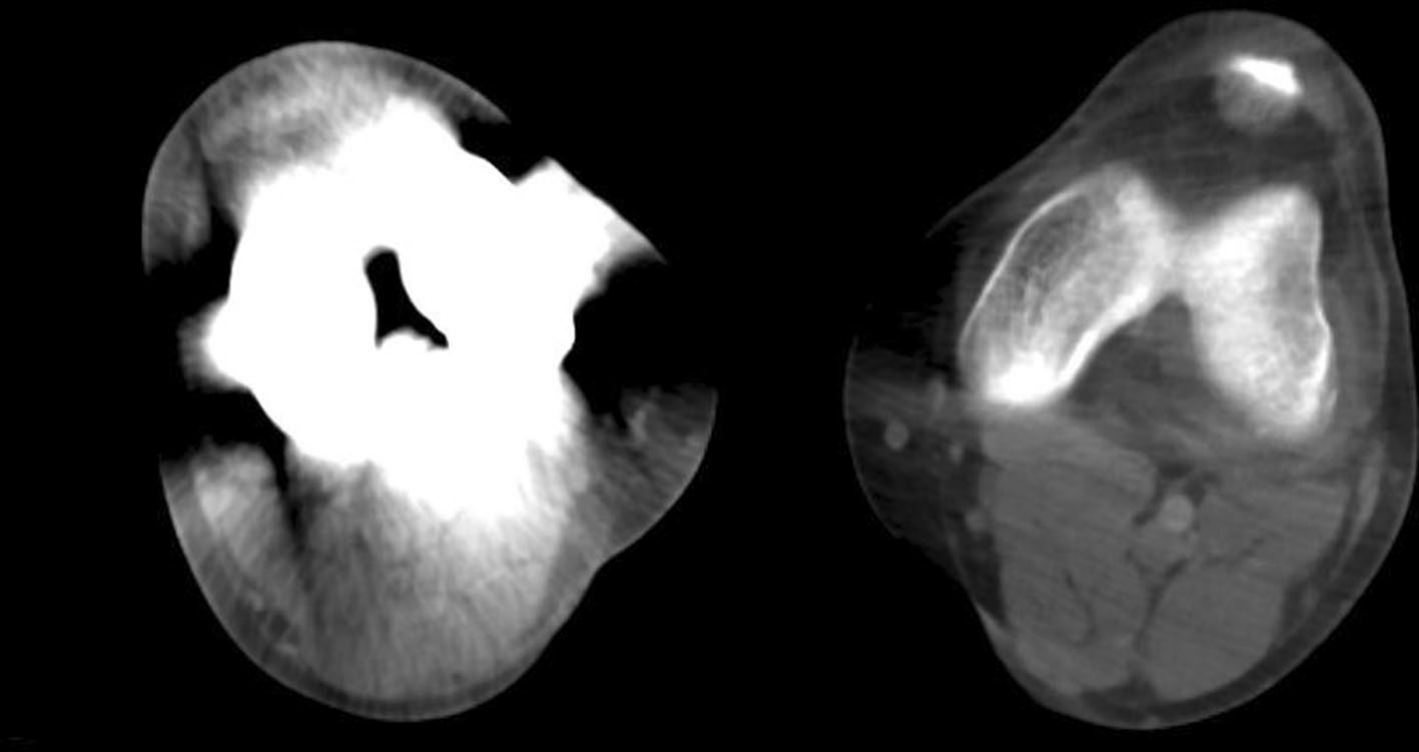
On postoperative computed tomography, assessment of the neurovascular structures near the knee joint gap is complicated by beam hardening artifacts around the metallic endoprosthesis
